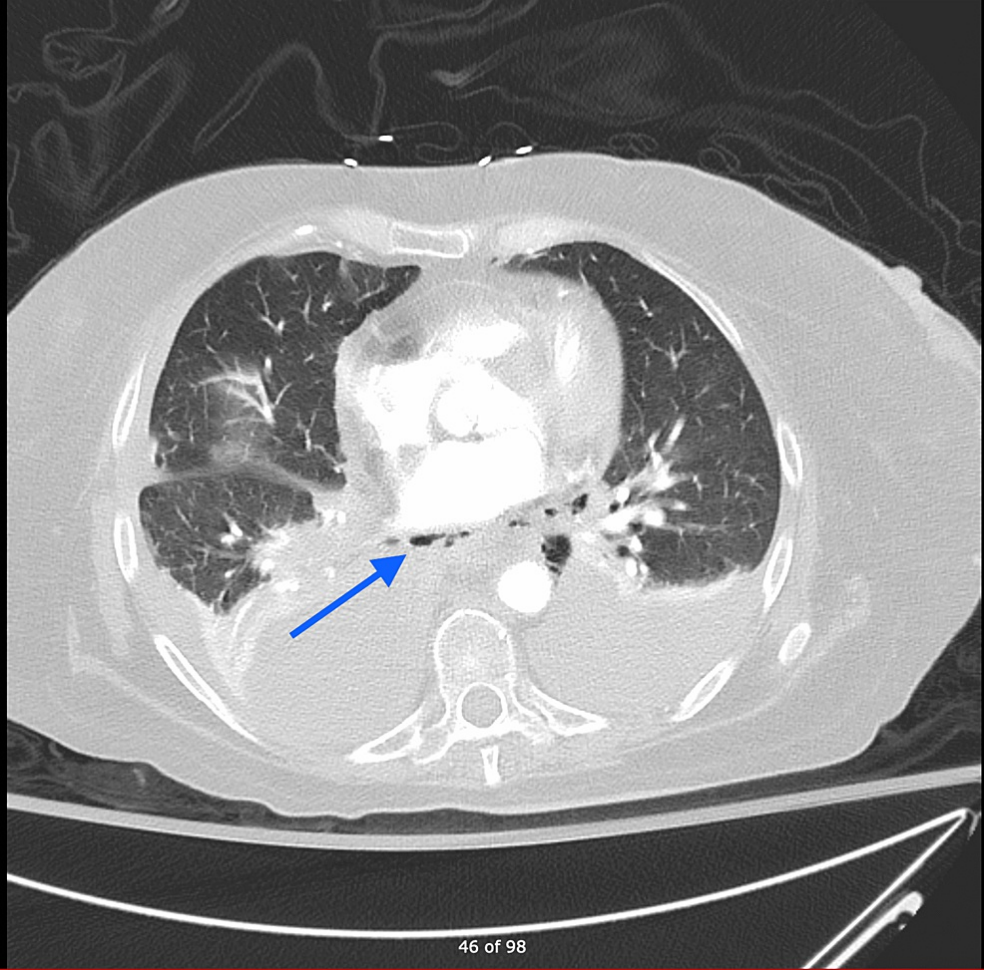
CTA chest showing pneumo-mediastinum. CTA: computed tomography angiography
